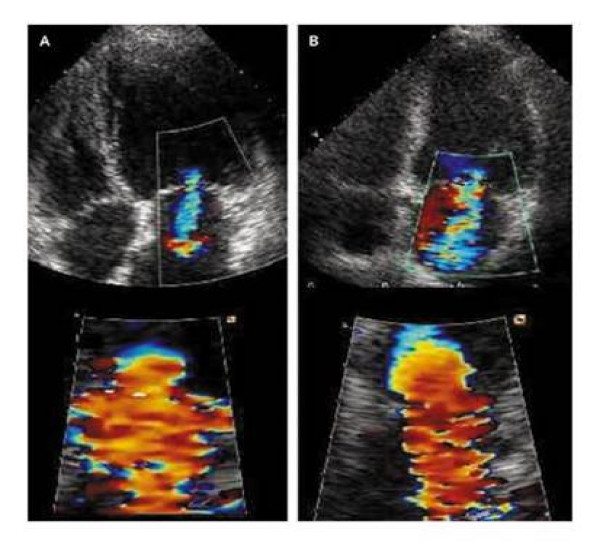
Color-flow Doppler echocardiogram and the flow convergence proximal to the ERO at rest (panel A) and peak exercise (panel B) in a patient with nonischemic pulmonary edema. From ref [10] A: rest. B: exercise.
